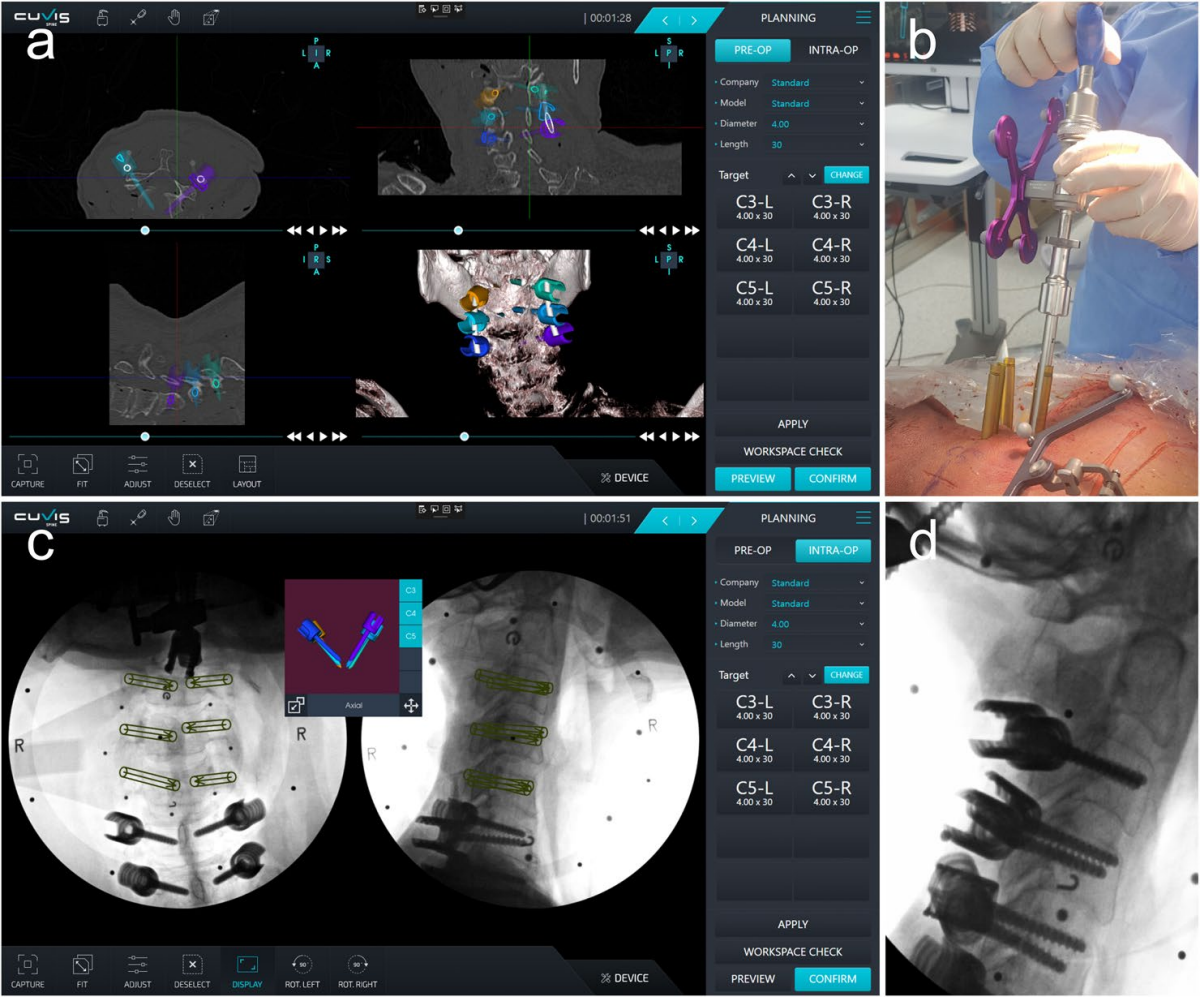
Procedures of robot-assisted posterior cervical pedicle screw insertion. (a) Preoperative CT-based planning of pedicle screw trajectory. (b) Robot-assisted pedicle screw placement. (c) Planned path overlaid on an intraoperative C-arm. (d) C-arm lateral image of cervical pedicle screw insertion.
